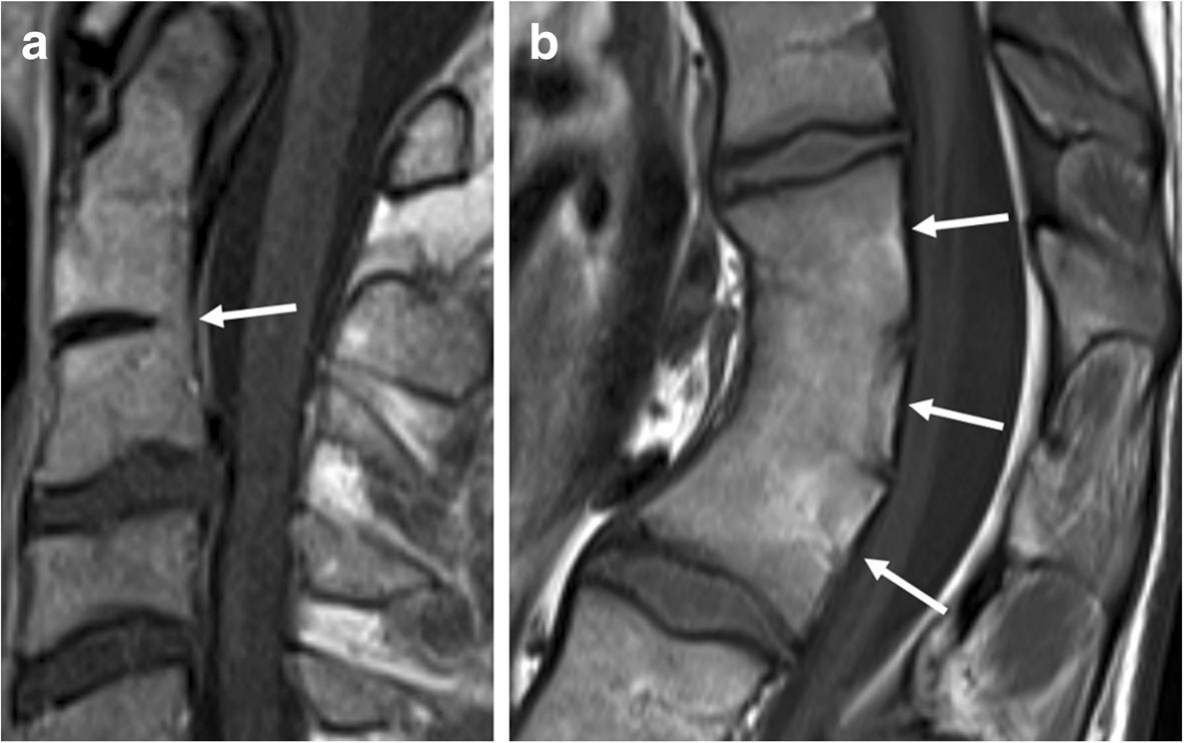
Pitfalls in imaging and diagnosis of new bone formation: congenital block vertebrae. Sagittal T1-weighted MR images show (a) partial congenital block vertebra of C2-C3 (arrow) and (b) complete congenital block vertebra consisting out of three vertebrae (arrows). Note the narrowed antero-posterior diameter in b, a typical sign of complete congenital fusion. Also, note that the height in b is less than the expected sum of three vertebrae and two intervertebral discs
